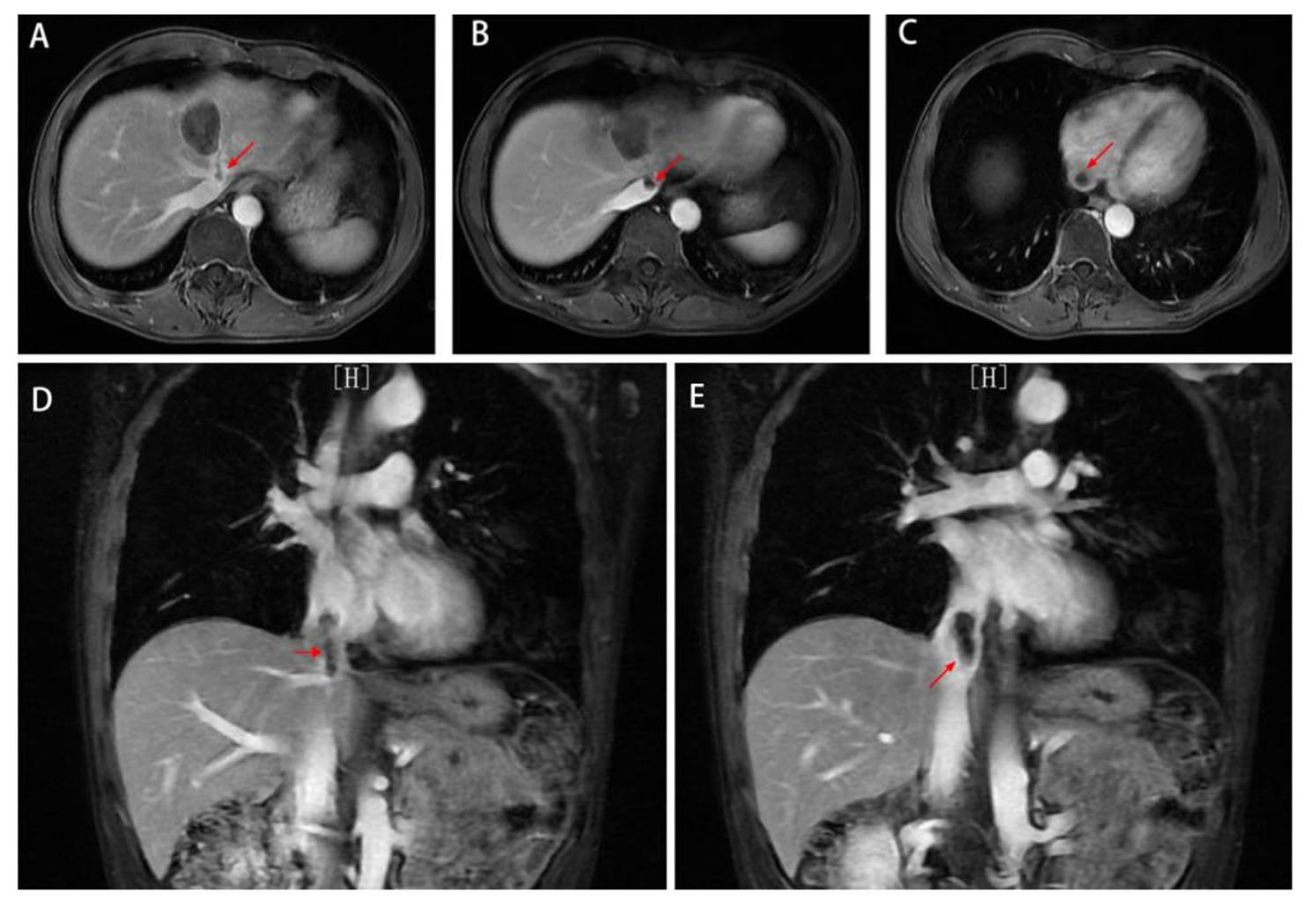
Imaging data from August 26th, 2022 A-E: MRI revealed the microwave ablation zone in S4; there was a thrombus(red arrow) in the left hepatic vein and IVC, and the head of the thrombus had entered the right atrium
